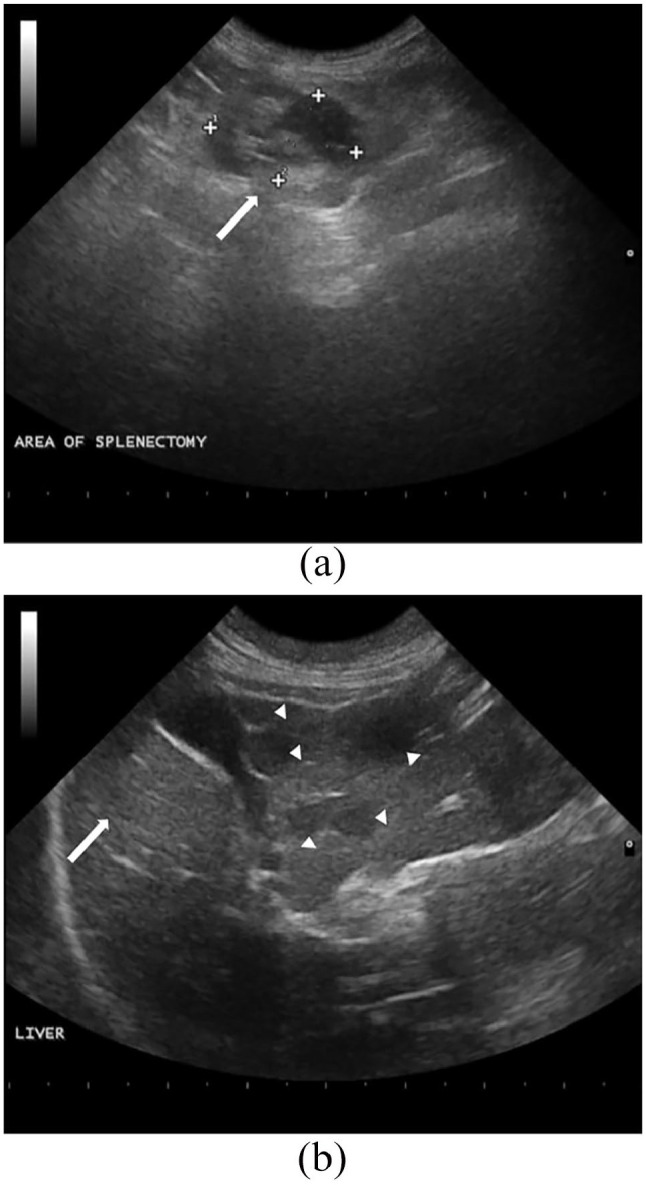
(a) Ultrasonographic image showing a lesion in the area of the previous
